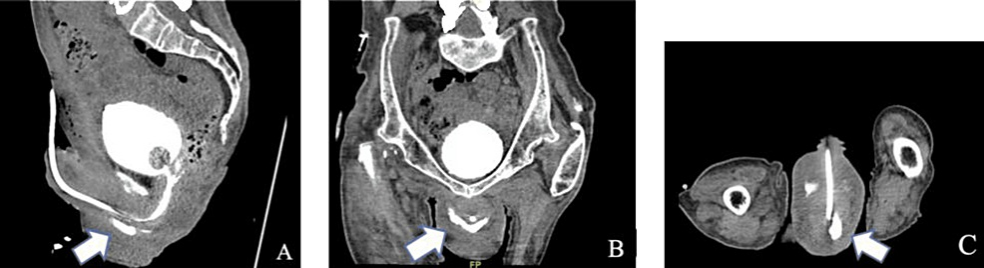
Sagittal (A), coronal (B), and transverse (C) views on CT cystography showing contrast filling the scrotum (arrow).
